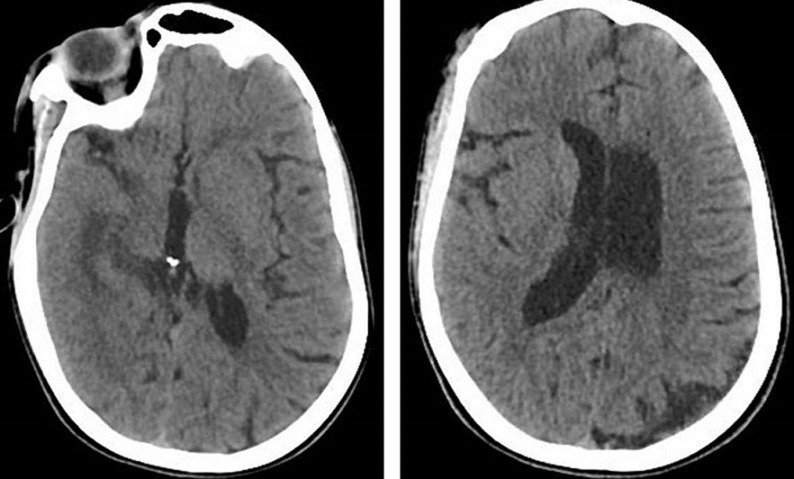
post-operative axial CT scan image; no collection and no lytic lesion
